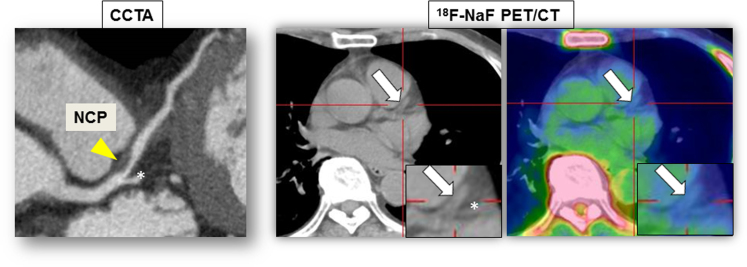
A 75-year-old male had a NCP in the proximal portion of the left anterior descending artery on CCTA (arrowhead). Its location on ^18^F-NaF PET/non-contrast CT (arrows) was determined using a vessel branch as a landmark (asterisks). The fused PET/CT image showed minimal ^18^F-NaF uptake corresponding to the NCP (TBR_max_ = 0.88).
